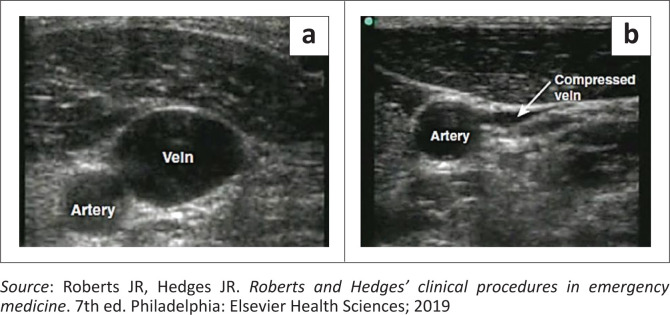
Ultrasound appearance of artery and vein. (a) Cross-sectional view of the artery and uncompressed vein. (b) Cross-sectional view of the artery and compressed vein.
